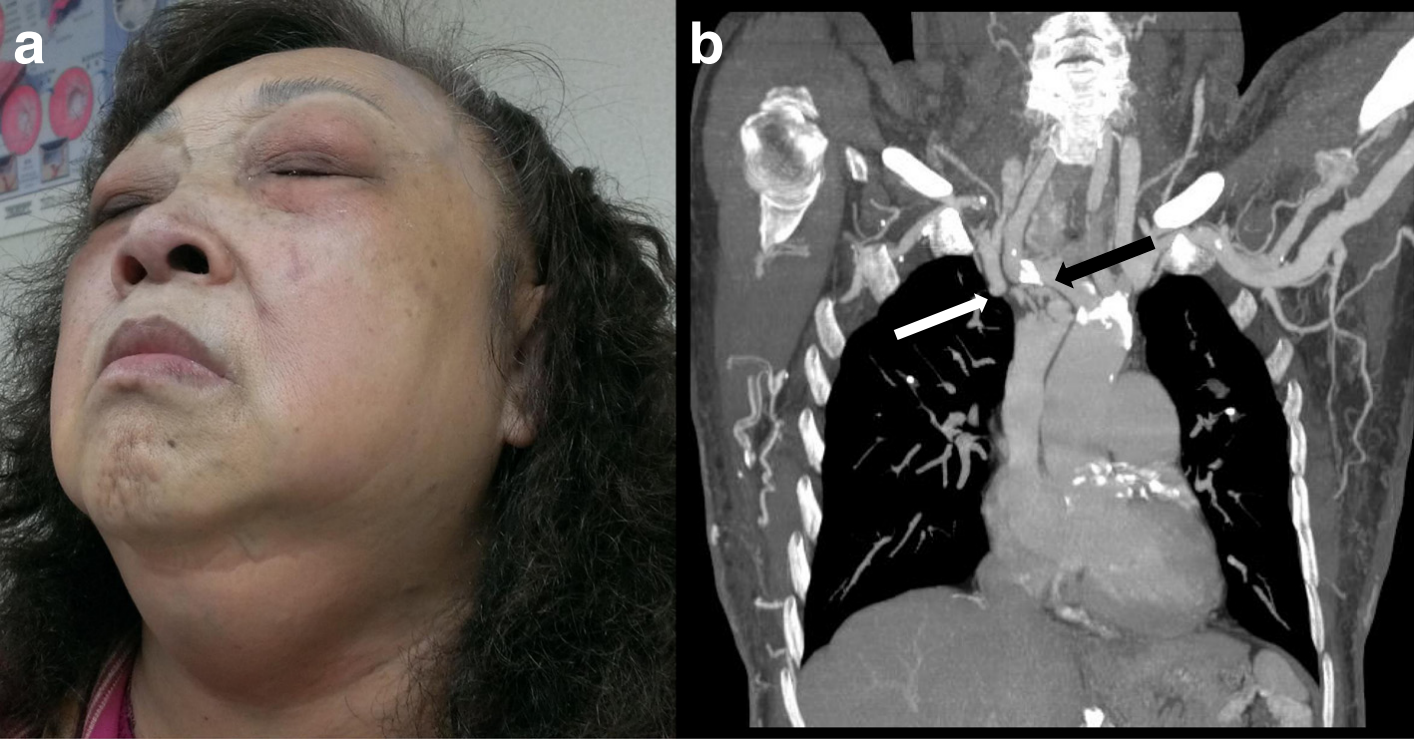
SVC syndrome occurred several times after repeated implantation of tunnelled double-lumen catheters for haemodialysis. a Gradual development of SVC syndrome with classic symptoms, including obvious face, neck, and upper trunk swelling. b A reconstructed coronal section of the contrast enhanced computed tomography angiogram revealed a fibrotic SVC. The occlusion (white arrow) of the right internal jugular vein at the junction with SVC and accompanying collateral blood-flow network (black arrow) indicated chronic SVC obstruction. (SVC = superior vena cava)
